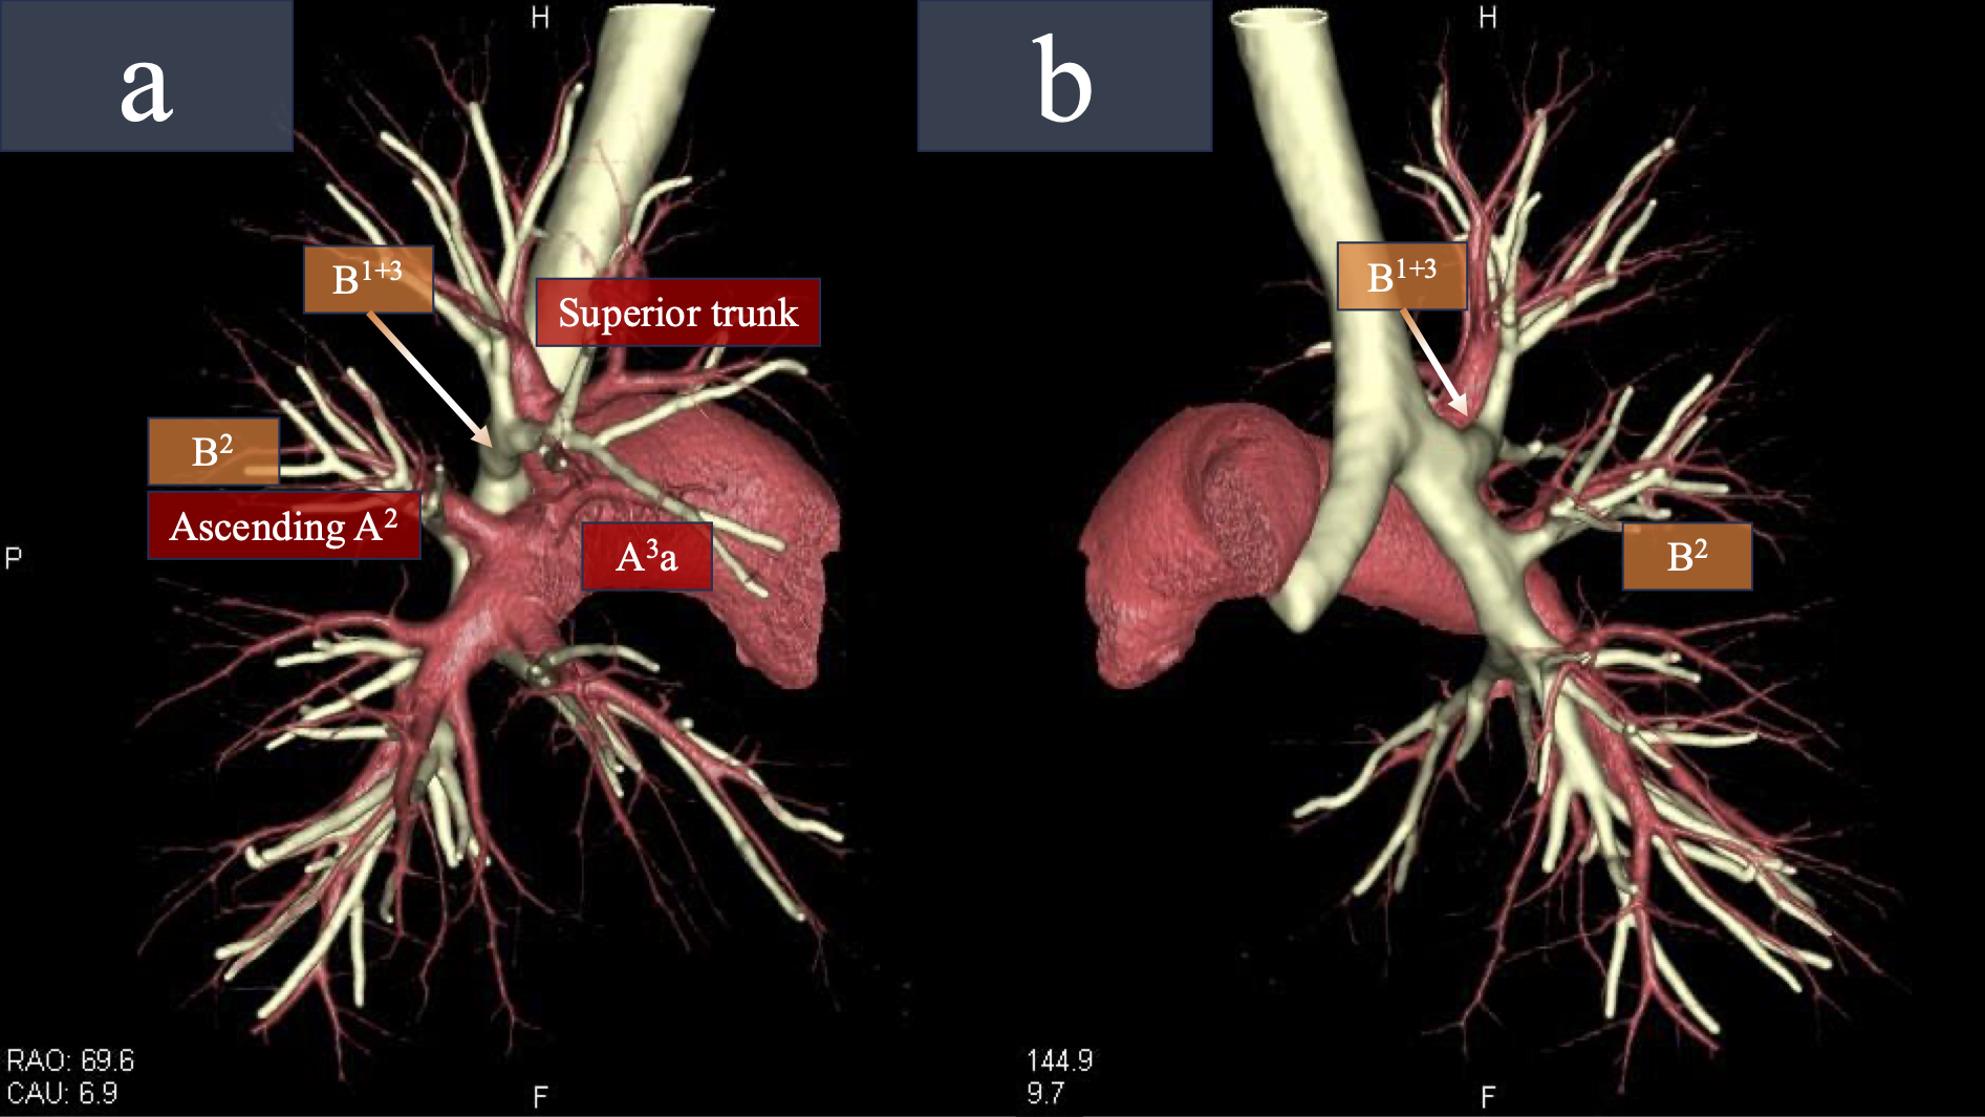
Three-dimensional CT bronchography and angiography (3D-CTBA), arterial phase, for preoperative anatomical planning (Ziostation REVORAS; Ziosoft Inc., Tokyo, Japan). a No recurrent A2 branch arose from the superior trunk (A1 + A3b). b The displaced B2 bronchus arising from the bronchus intermedius was clearly visualized
